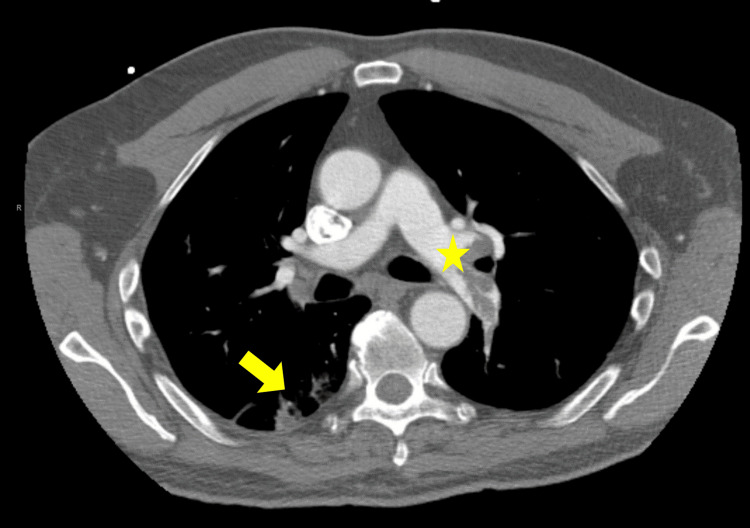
Transverse CT scan with contrast showing filling defect (star) in the left pulmonary arteries with right posterior pulmonary infarct (arrow).
